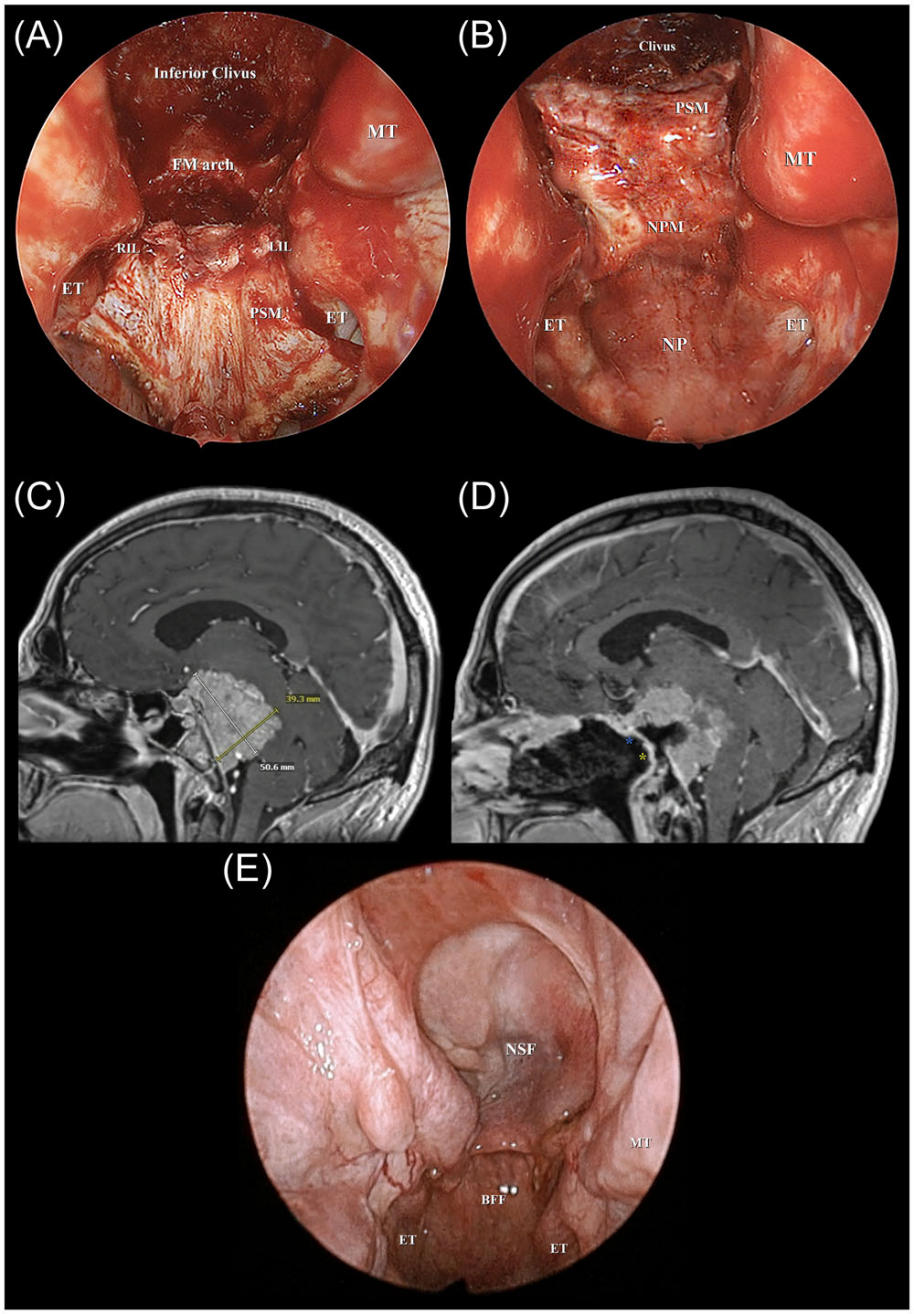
Shows a case of petroclival meningioma. (A) An endoscopic endonasal view showing BFF incisions. (B) An endoscopic endonasal view showing BFF rotated postero‐superiorly towards the clivus. (C) Preoperative T1 weighted MRI with contrast showing the petroclival meningioma measuring about 3.9 × 5.1 cm and compressing the brain stem. (D) Postoperative T1 weighted MRI with contrast showing central tumor debulking and reconstruction with the nasoseptal flap superiorly (blue asterisk) and the butterfly flap inferiorly (yellow asterisk) overlapping at mid‐clivus, both flaps show contrast enhancement. (E) An endoscopic endonasal view showing healing of both nasoseptal and butterfly flaps 1 month postoperatively. BFF, butterfly flap; ET, eustachian tube; FM arch, foramen magnum arch; LIL, left incisional line; MT, middle turbinate; NP, nasopharynx; NPM, nasopharyngeal mucosa; NSF, nasoseptal flap; PSM, posterior septal mucosa; RIL, right incisional line.
